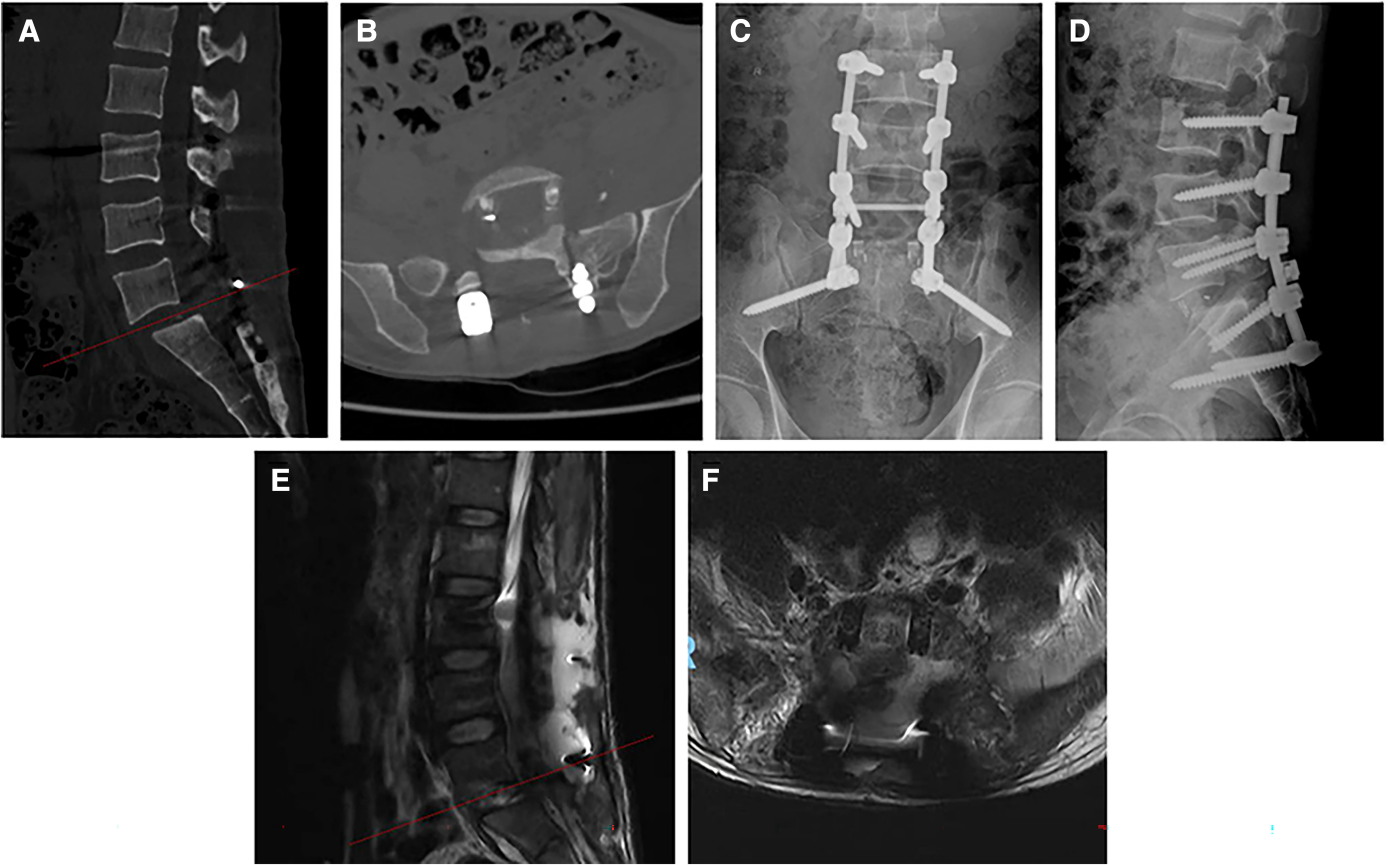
Sagittal and cross-sectional CT images at the postoperative month 1 (A,B). Lumbar anteroposterior and lateral x-ray radiographs at the postoperative month 1 (C,D). Sagittal and cross-sectional MRI images at the postoperative month 1 (E,F). CT, computed tomography; MRI, magnetic resonance imaging.
